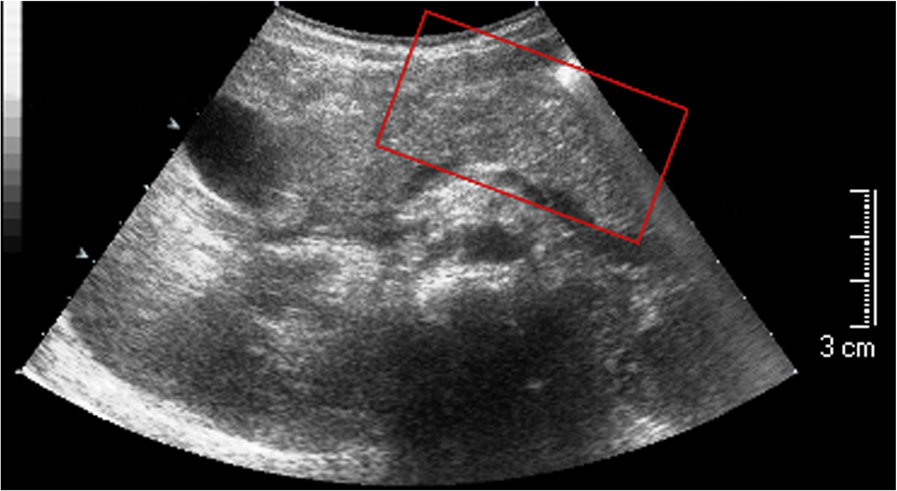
Ultrasound scan showing the pancreas increased in size owing to a large edematous area involving the pancreatic head and tail.
